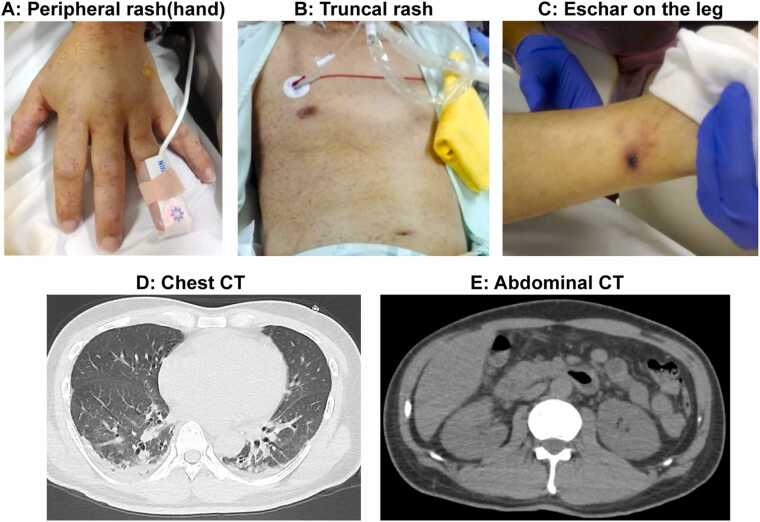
Clinical photographs. A: Peripheral rash (hand). B: Diffuse truncal rash. C: Eschar on the lower leg. D: Chest CT. E: Abdominal CT.
